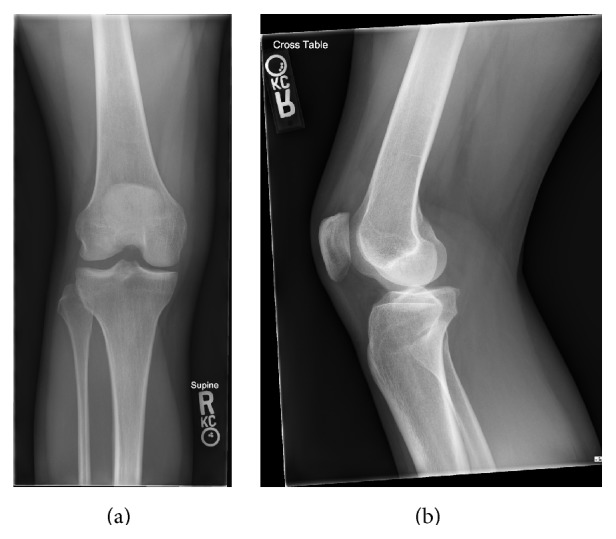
AP (a) and lateral (b) view radiographs of right knee for the evaluation of acute injury. Lateral view demonstrates moderate joint effusion, deepening of the terminal sulcus, and irregularity of the posterior aspect of the lateral tibial plateau.
